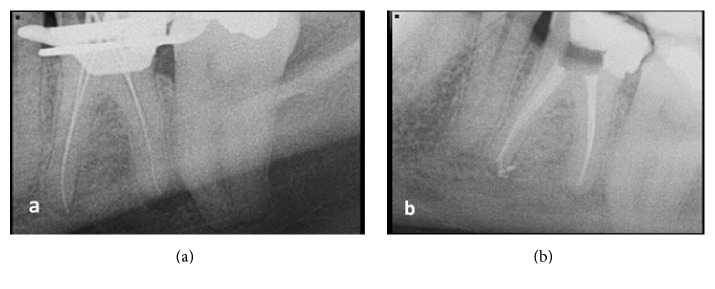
(a) Radiograph for working length confirmation: case report number 2. (b) Final radiograph: case report number 2.
